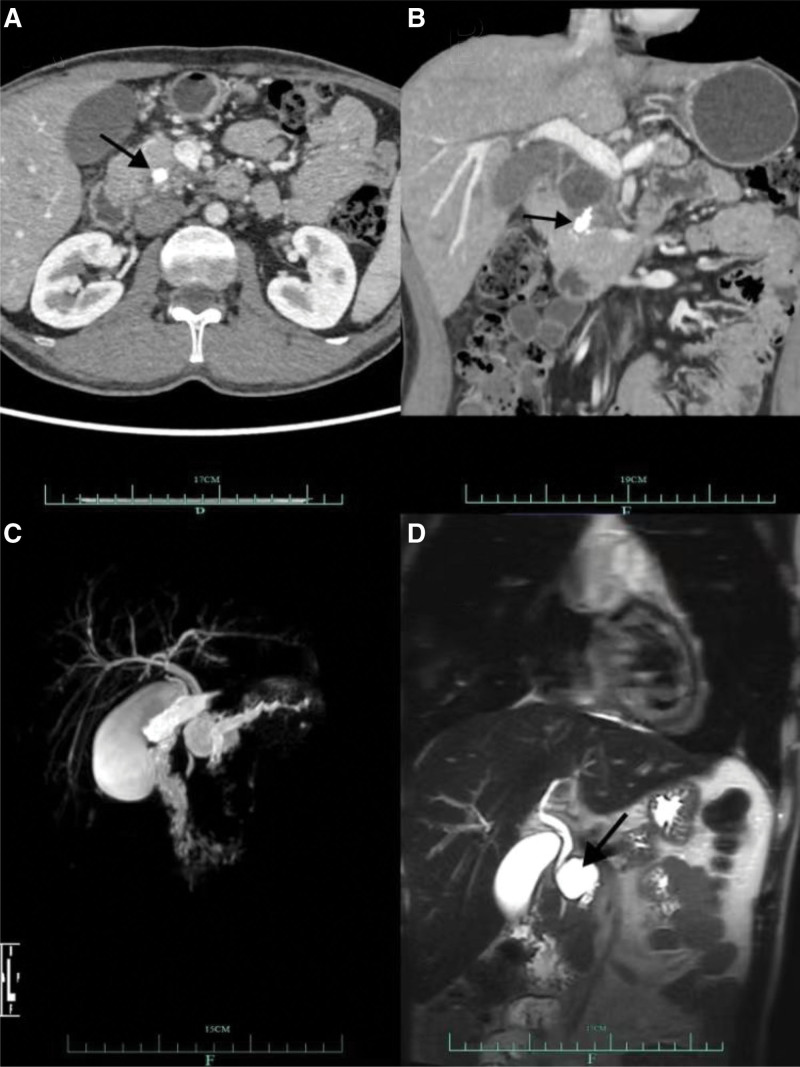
(A) High-density intraductal calculus in the pancreatic head region observed on computed tomography. (B) Pancreatic duct stones and cysts located above the head of the pancreas are visible in the coronal plane. (C) Magnetic resonance cholangiopancreatography revealed significant thickening of the pancreatic duct and cysts in the head region of the pancreas. (D) Dilatation of the pancreatic duct and cysts in the head of the pancreas.
